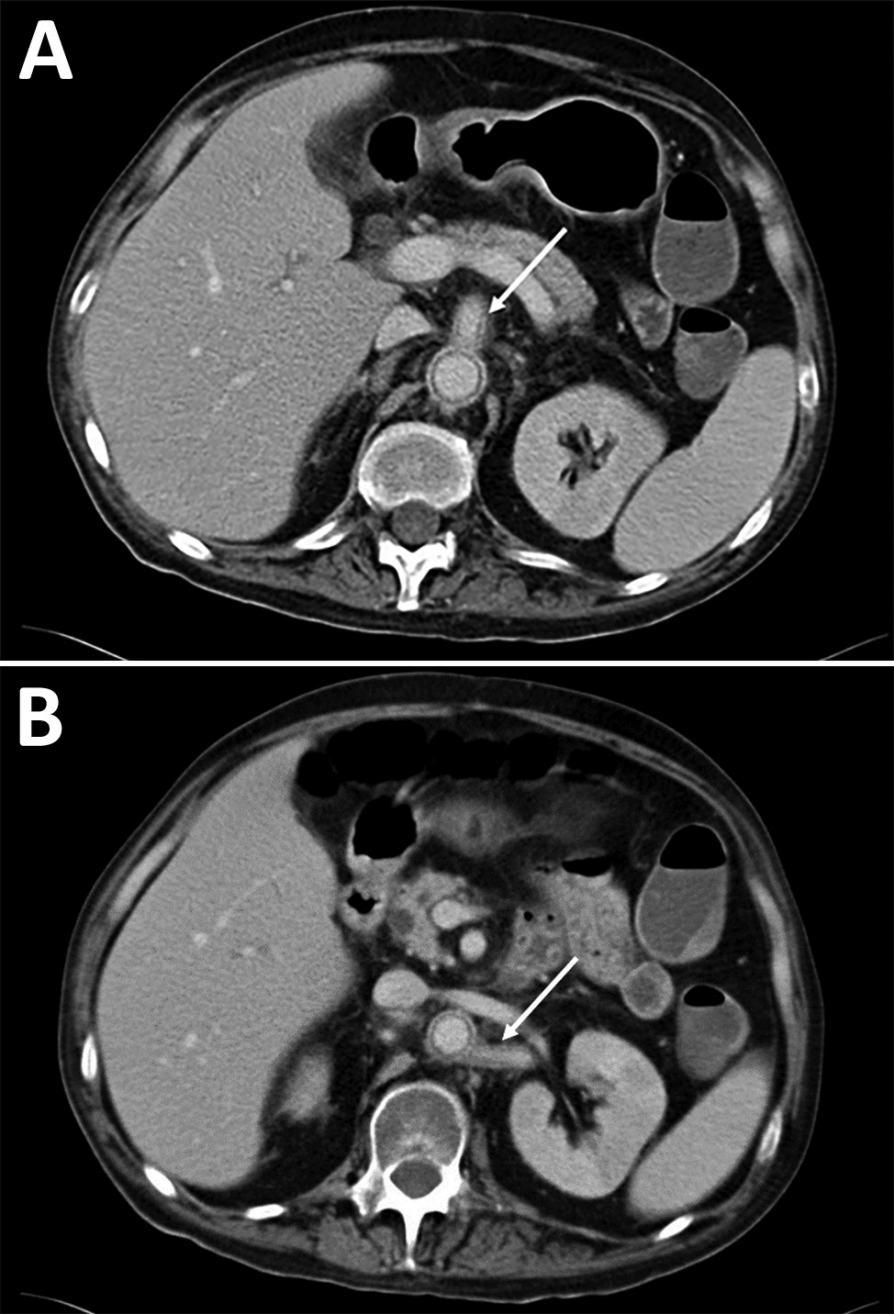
Abdominal computed tomography scans for case-patient 5 showing aortitis in study of unexpected vascular locations of *Scedosporium* spp. and *Lomentospora prolificans* fungal infections, France. A) Arrow indicates abdominal aorta and superior mesenteric artery thickening and perivascular contrast. B) Arrow indicates abdominal aorta and left renal artery thickening and perivascular contrast. Data are from the Scedosporiosis/lomentosporiosis Observational Study (*12*).
